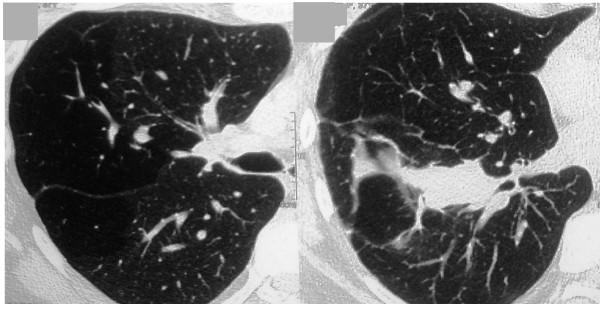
Imaging after treatment. Computed tomography scan demonstrates a decrease in the lesion size.
